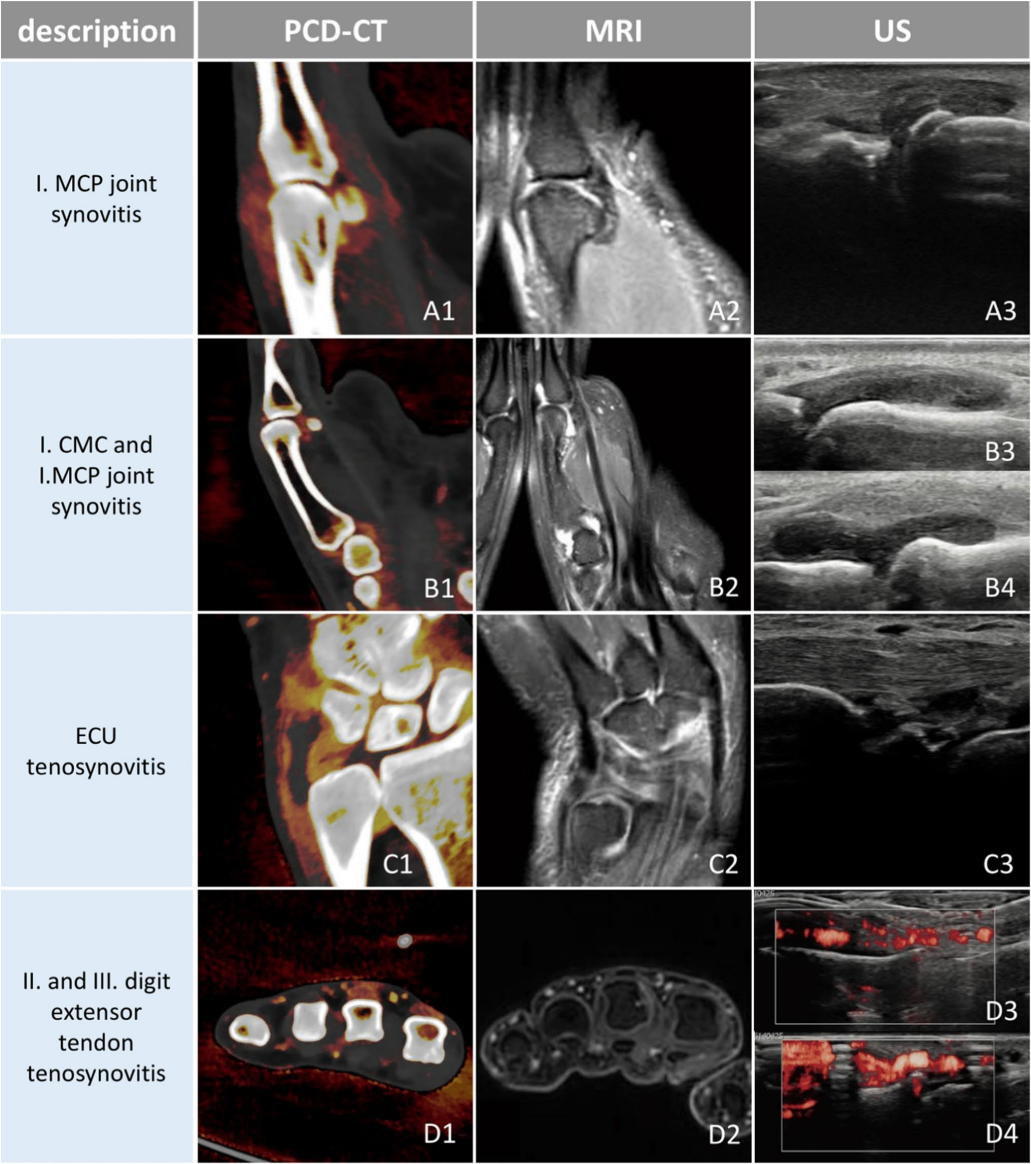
Comparisons of common pathologies in the same patients across different modalities used in the investigation of peripheral arthritis. On PCD-CT iodine maps, synovitis and tenosynovitis appear as areas of thickened synovium and tenosynovium with high iodine concentration, represented in red on the color map. On PD MRI sequences (A2, B2, C2), these pathologies present as high-signal fluid within the joint capsule or around the tendon, thickened synovium with intermediate signal intensity, and enhanced contrast uptake on post-contrast sequences (D2). In some cases, inflammation is more conspicuous on iodine maps than on contrast-enhanced MRI, particularly in distal digital structures, due to higher spatial resolution, improved contrast, and reduced motion artifacts (D1 and D2). Ultrasound reveals intraarticular and peritendineal fluid accumulation, thickened synovium, and hypervascularity, which is highlighted on Doppler imaging. PCD-CT iodine maps provide a comprehensive and standardized overview of the affected hand while delineating inflammation with great contrast and high resolution, minimizing study duration and artifacts and allowing for the sensitive detection of bony changes at the same time. CMC, carpometacarpal; ECU, extensor carpi ulnaris; IP, interphalangeal; MCP, metacarpophalangeal; MRI, magnetic resonance imaging; PCD-CT, photon-counting detector CT; PD, proton density; US, ultrasound
